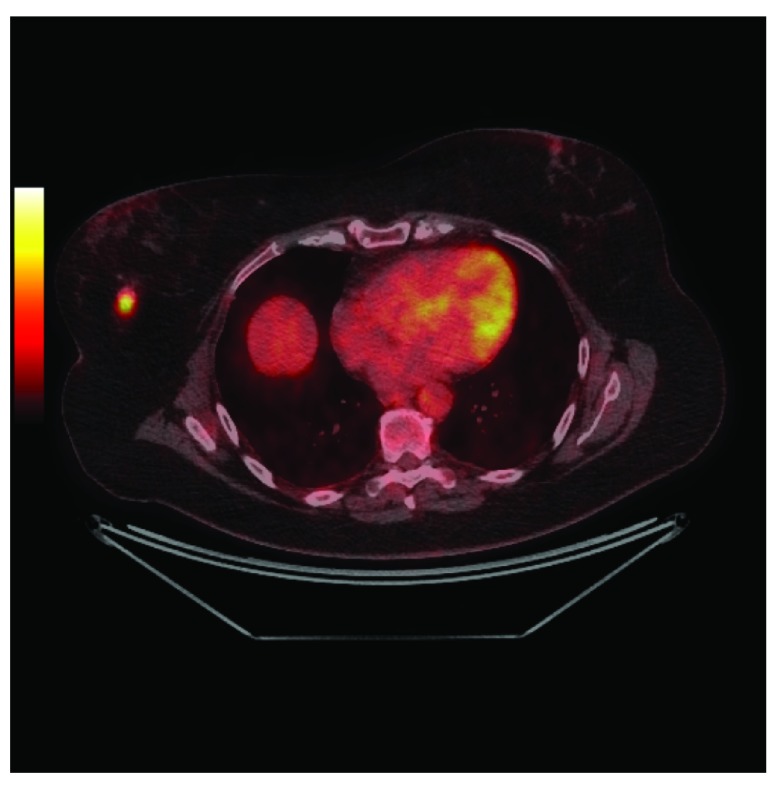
FDG PET/CT image of the breast metastasis. Fused FDG PET/CT images showing an intense focal FDG uptake in the right breast coinciding with a dense breast nodule on CT images. The hot iron FDG PET scale represents intensity of FDG uptake, varying from black at the weakest intensity to white at the strongest.
